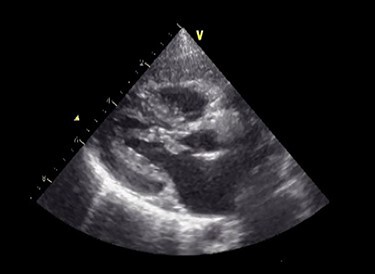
Preoperative echocardiography. The abnormal muscle band in left ventricle and severe SAM of the mitral valve are observed. The anterior leaflet of mitral valve obstructs the LVOT in the systolic phase.
